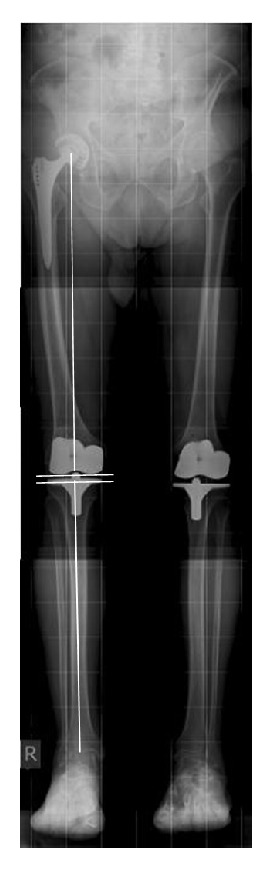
Long leg standing X-ray performed in anterior-posterior (a.p.) view. The mechanical axis, the distal lateral femur angle, and the proximal medial tibial angle of the TKA were measured.
